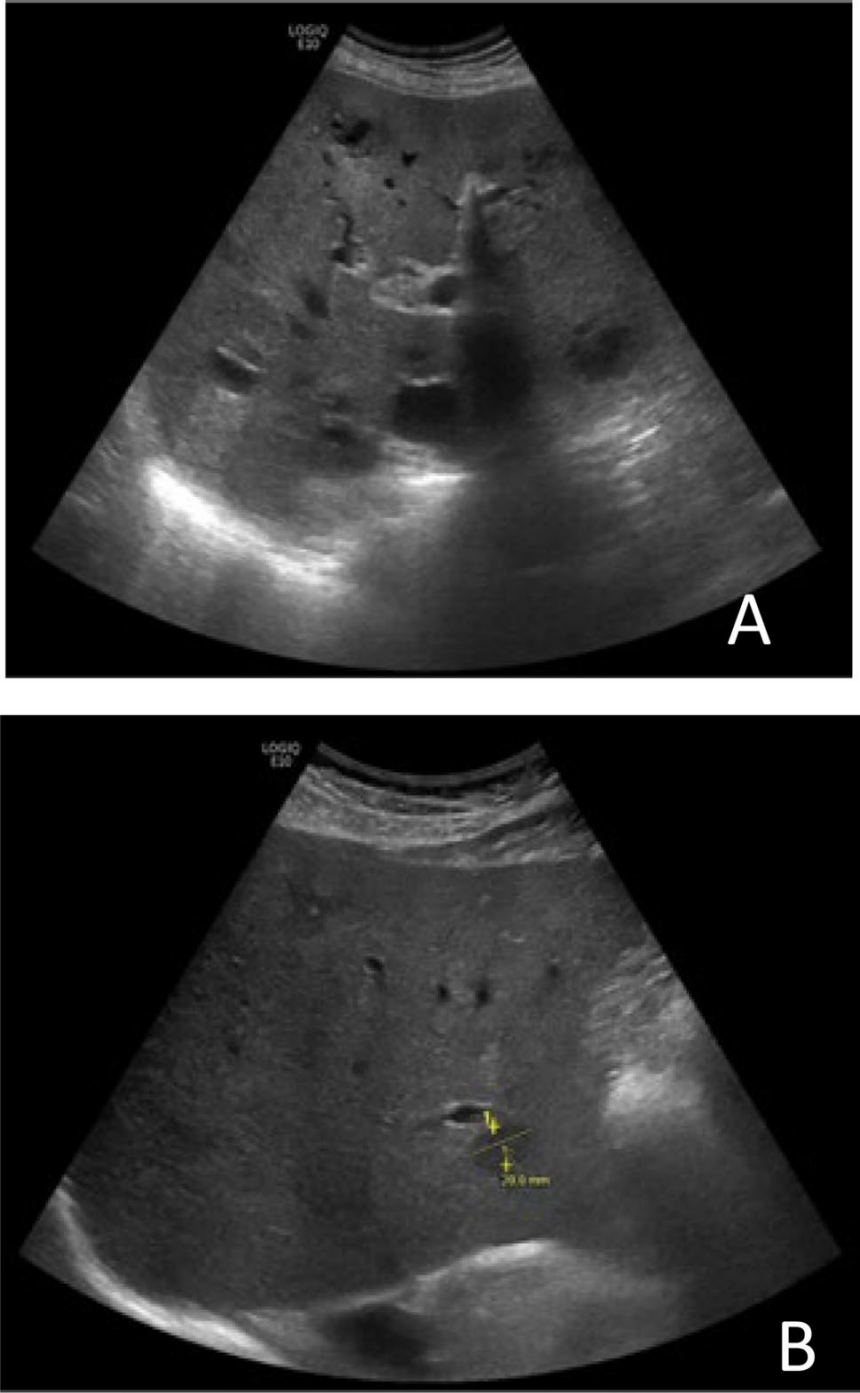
Ultrasound shows multiple liver abscesses (A), and residual abnormalities at the place of previous liver abscessed (B)
